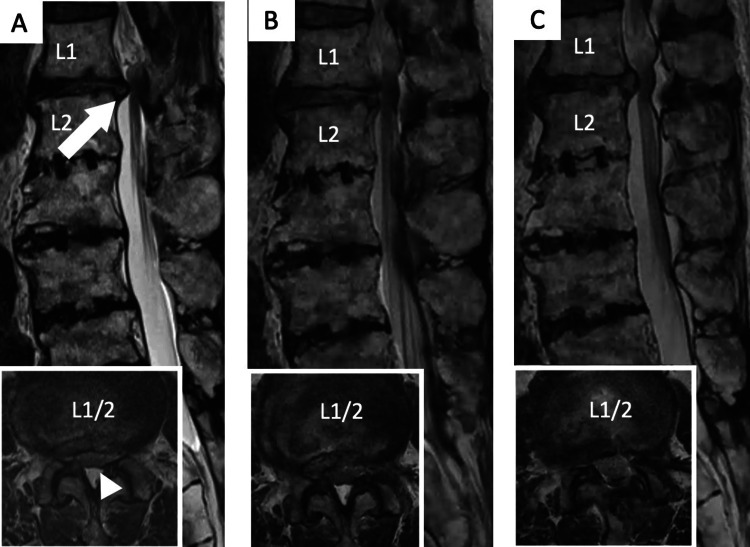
Case 3: A 65-year-old male with radiating pain in his lower extremities, predominantly on the left side. (A) Preoperative MRI revealing a central-type disc herniation (arrow) with spinal canal stenosis (arrowhead) compressing the dural sac at L1–L2. (B) MRI after full endoscopic lumbar discectomy from the left side via transforaminal approach. (C) MRI after additional full endoscopic lumbar laminectomy from the left side via the interlaminar approach. (This case is original and has not been previously reported.)
